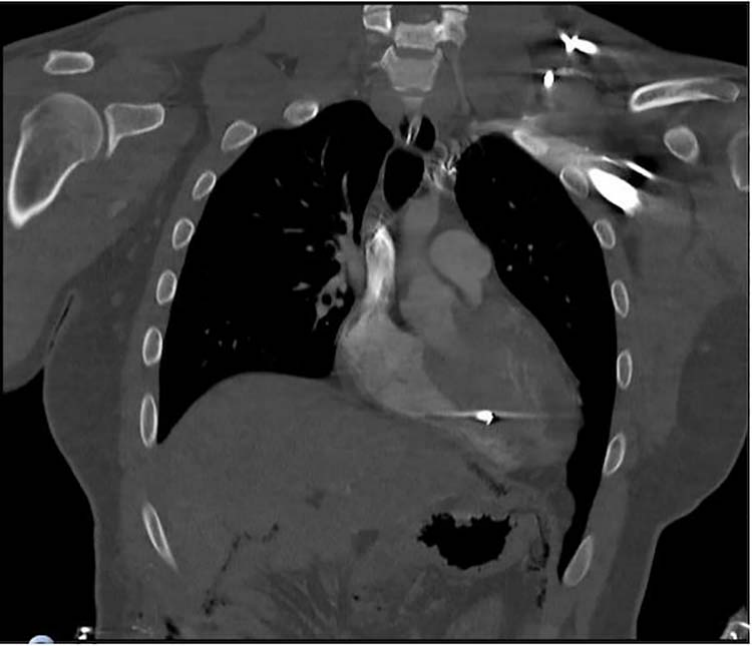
Coronal CT scan of the chest identifying bullet fragment in the interventricular septum.
